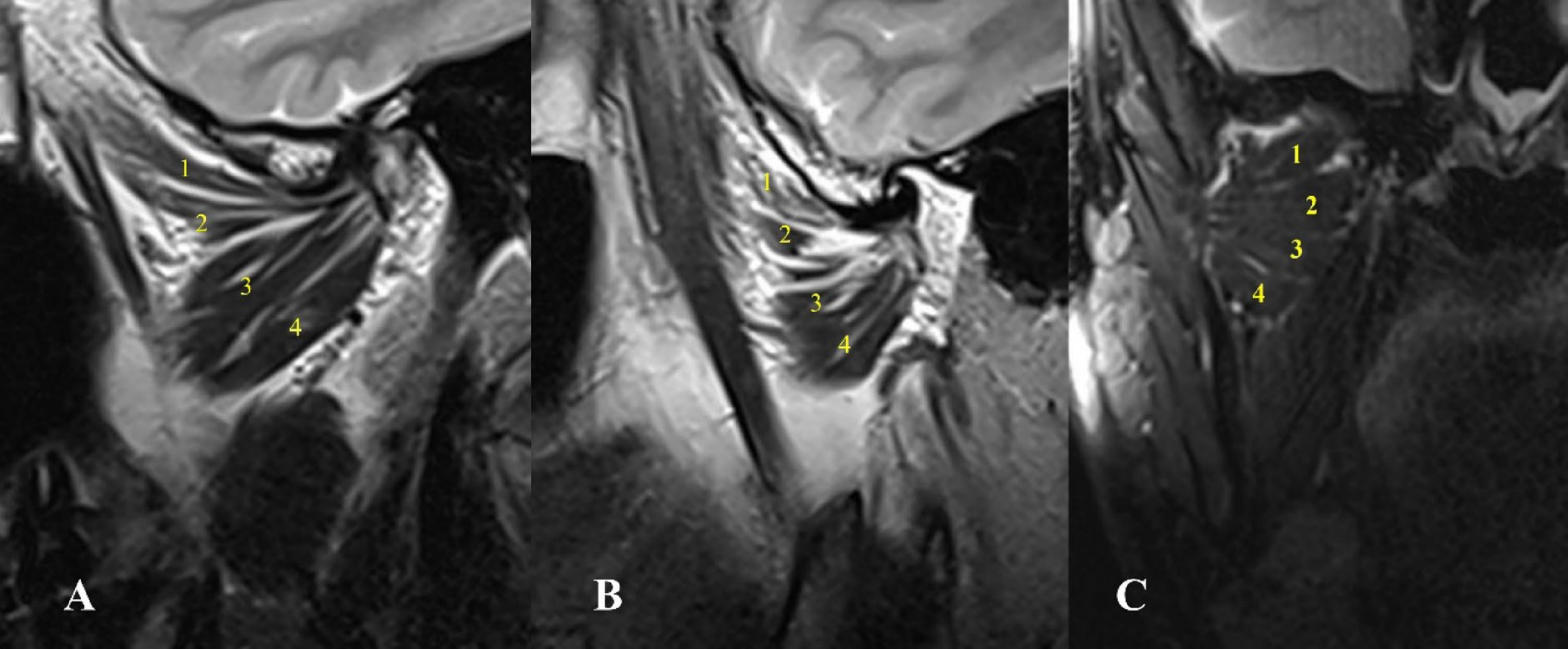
Oblique sagittal T1-weighted images represent Type IV with four heads of lateral pterygoid muscle, the upper attached to the disc and the lower three attached to the condyle associated with condylar erosion, and condylar resorption.
